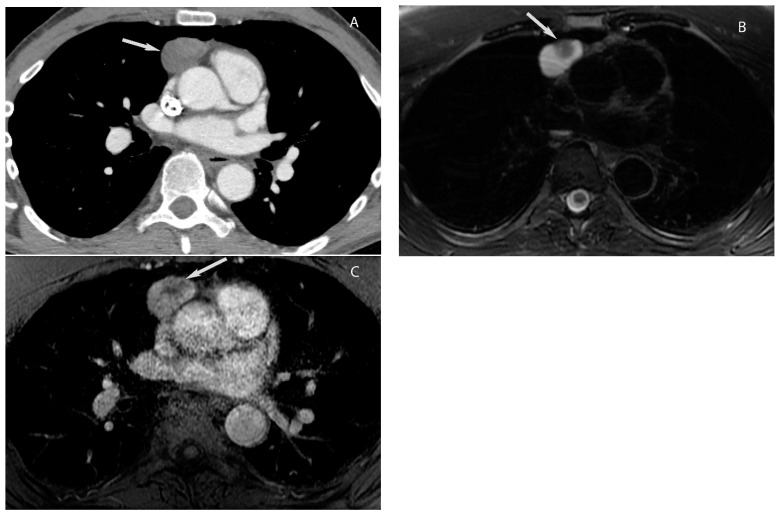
Cystic thymoma. CT (A) shows a mixed attenuation (cystic and solid) mass in the prevascular mediastinum. T2W MRI (B) shows hyperintense cystic component and hypointense solid nodule (arrow in (B)). Post-gadolinium MRI (C) shows enhancement of the solid nodule. Pathology showed cystic thymoma.
